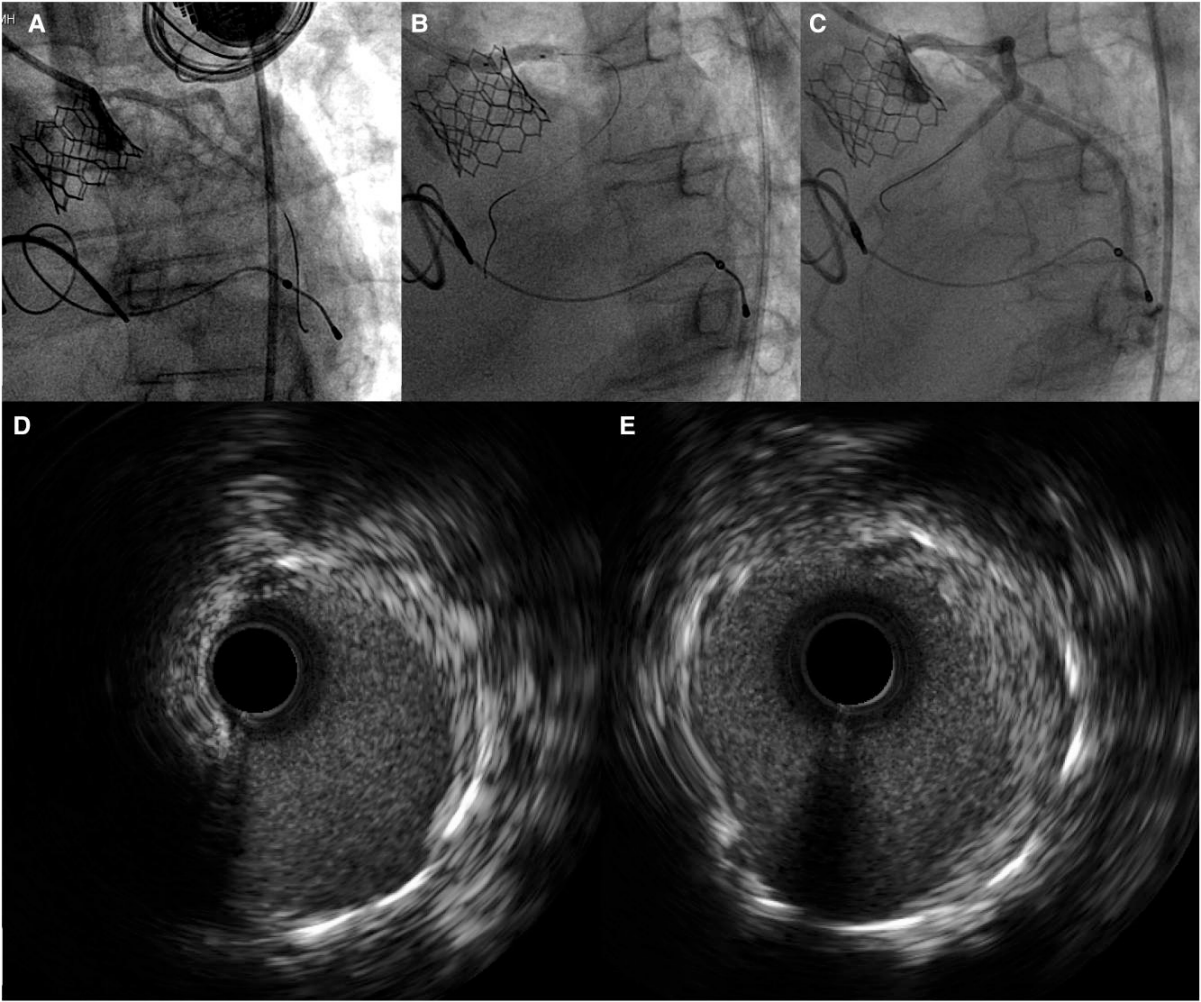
In-stent restenosis percutaneous coronary intervention to the left main chimney stent. In-stent restenosis of chimney stent inside neosinus (*A*). Post POBA, including cutting balloon dilatation and drug-coating balloon over chimney stent at the left main (*B*). Regain the potency of chimney stent in left main in final angiogram (*C*). The intravascular ultrasound image of stenosis of chimney stent post CB and DCB dilatation (*D* and *E*) with image (*D*) position correlating to *Figure 3B* and image (*E*) to *Figure 3C*.
